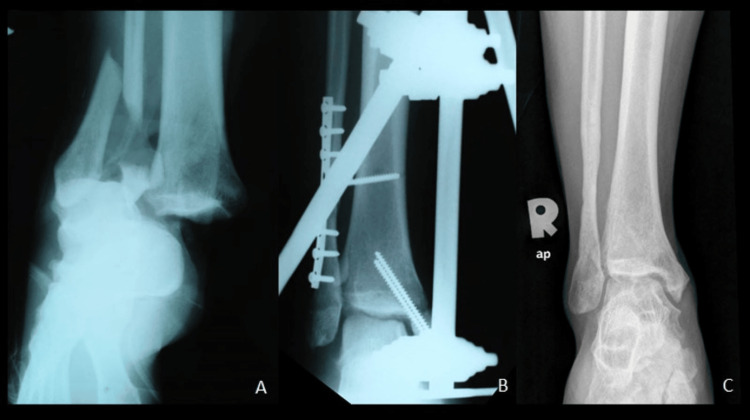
Radiograph during injury showing right ankle bimalleolar fracture with tibiotalar joint dislocation (A), post internal fixation with temporary cross ankle external fixator (B). Radiograph at one year of trauma showed union of bimalleolar fracture with osteochondral injury at anteromedial talar dome (C).
